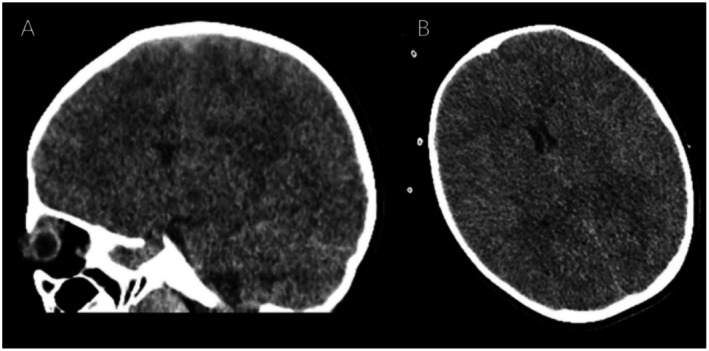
Cranial computed tomography showing a diffuse decrease in brain parenchymal density. (A) Sagittal view; (B) Transverse view.
